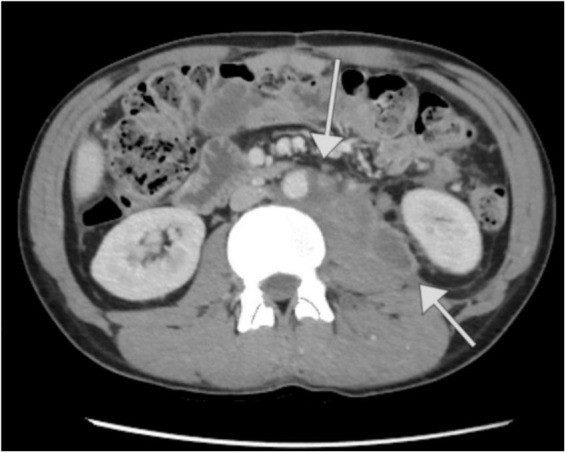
Computed tomography image of the patient’s abdomen after completion of chemotherapy performed at The Maria Sklodowska-Curie National Research Institute of Oncology in Warsaw. Partial tumor regression.
